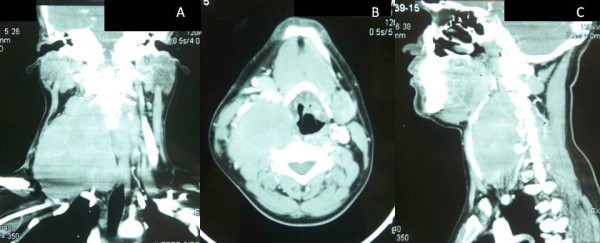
Coronal (A), axial (B) and sagittal (C) CT scans with contrast showing the mass measuring about 9.3 × 5.9 × 12 cm with areas of central necrosis. The carotid sheath is displaced anteriorly.
